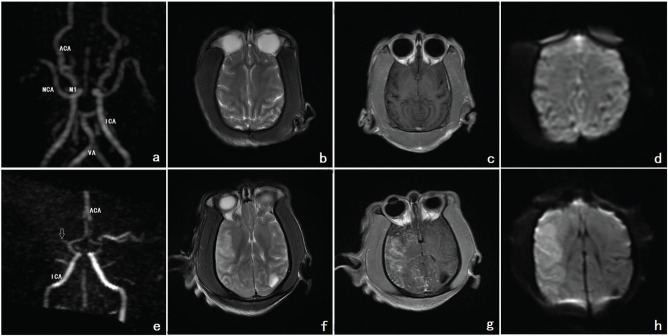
a, b, c, d Before the operation, MRA showed the MCA and its segments. The ACA, ICA and VA can also be seen. On T2, contrast-enhanced T1 and DWI imaging, the normal cerebral parenchyma of the MCA showed iso-signal intensity. e, f, g, h After the operation, MRA observed that the MCA and its segments were occluded. The occlusion is indicated by the white arrow. On T2, contrast-enhanced T1 and DWI imaging, the MCA demonstrated high signal intensity. Cerebral infarction formation in the parenchymal area was revealed. Footnote: MRA refers to images a and e; T2 refers to images b and f, contrast-enhanced T1 refers to images c and g; DWI refers to images d and h (MRA=magnetic resonance angiography; MCA=middle cerebral artery; ACA=anterior cerebral artery; ICA=internal carotid artery; VA=vertebral artery; DWI= diffusion-weighted imaging).
